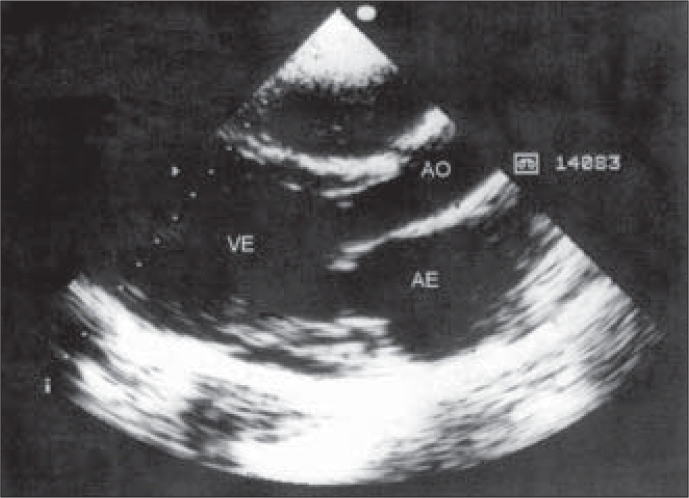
Echocardiogram showing outlet of left ventricle with dilated chambers.
